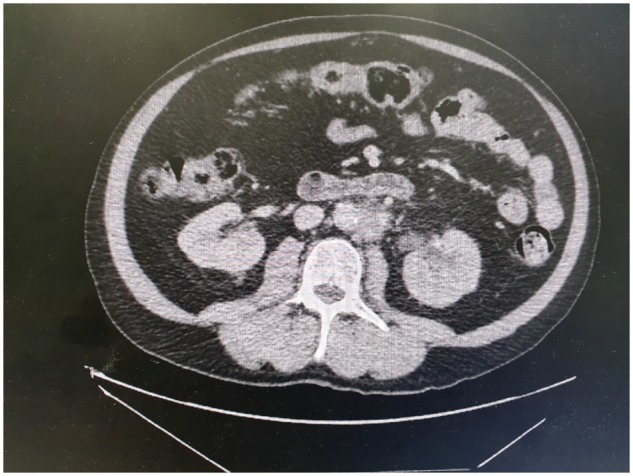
CT scan with contrast (Case 3), showing periaortic soft tissue
